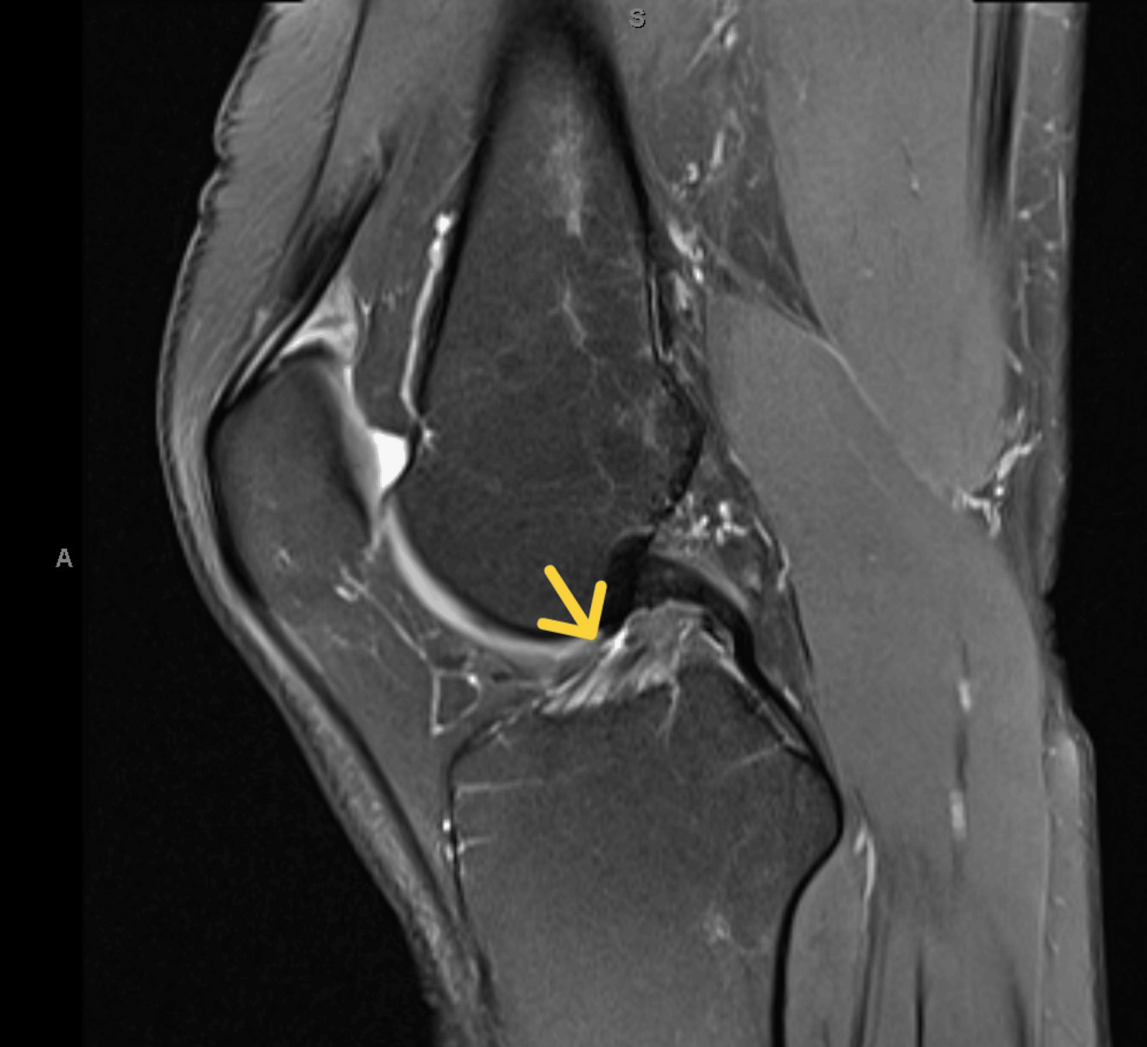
Lateral MRI of ACL exhibiting 10% tear ACL, anterior cruciate ligament; MRI, magnetic resonance imaging
